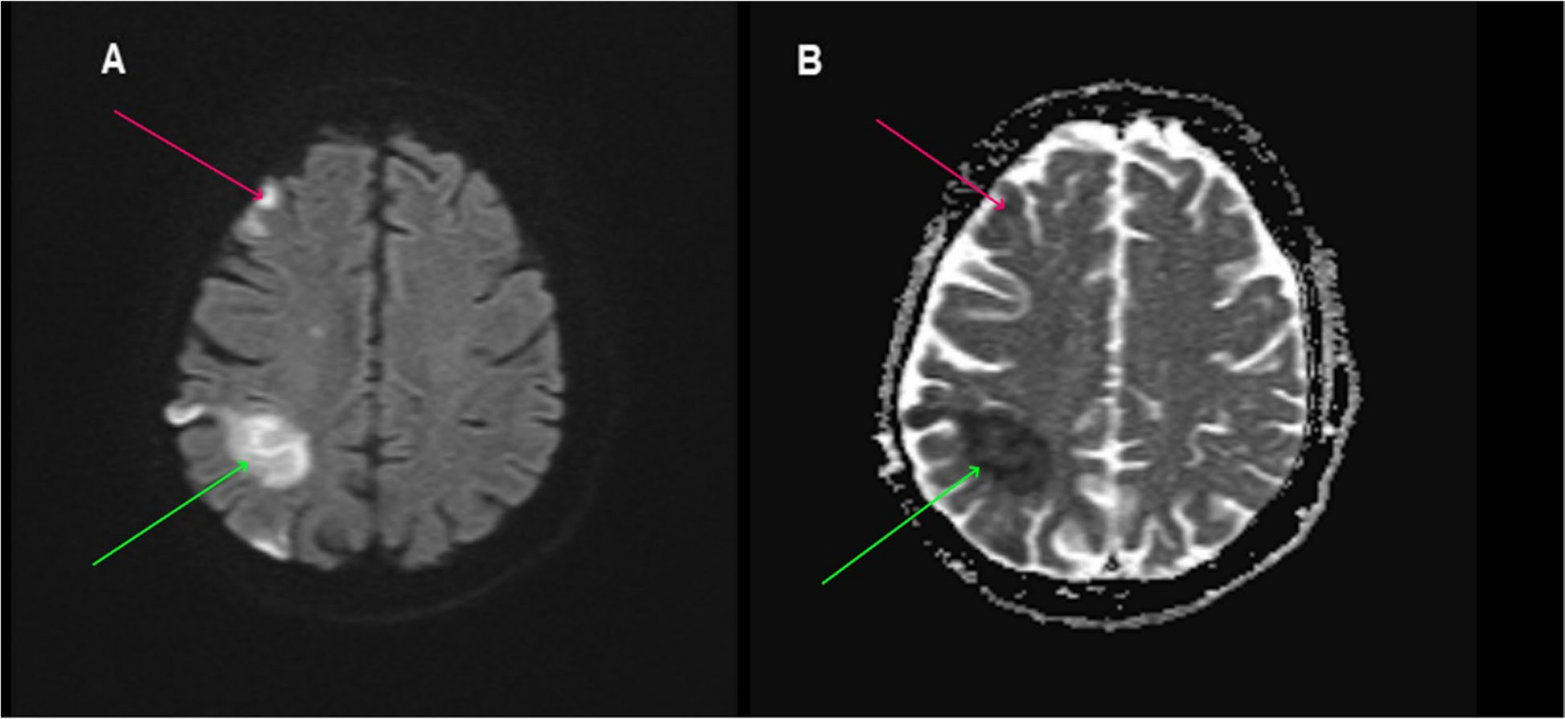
A Diffusion-weighted magnetic resonance imaging axial section of the brain showing restricted diffusion in the right frontal lobe (red arrow) and right parietal lobe (green arrow). B Apparent diffusion coefficient (ADC) magnetic resonance imaging axial section of the brain showing low ADC values in the right frontal lobe (red arrow) and right parietal lobe (green arrow)
